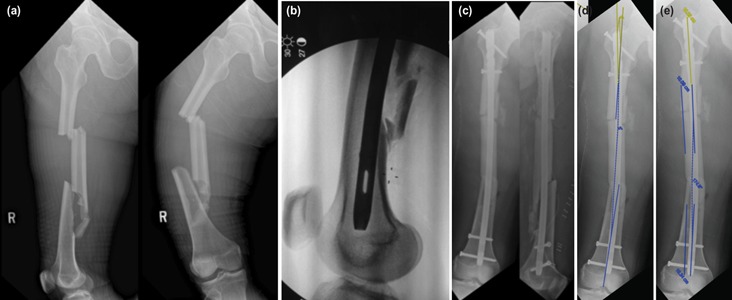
(a) Preop radiograph of right femoral segmental fracture, (b) Intraop fluoroscopic lateral knee view: two distal screws are parallel with lateral knee axis, (c) immediate post op radiograph anterior posterior view and (d) lateral view, (e) 5° valgus malalignment between proximal and distal femoral segments, 5.4° valgus malalignment between middle and lower third portion of the A2FN nail.
